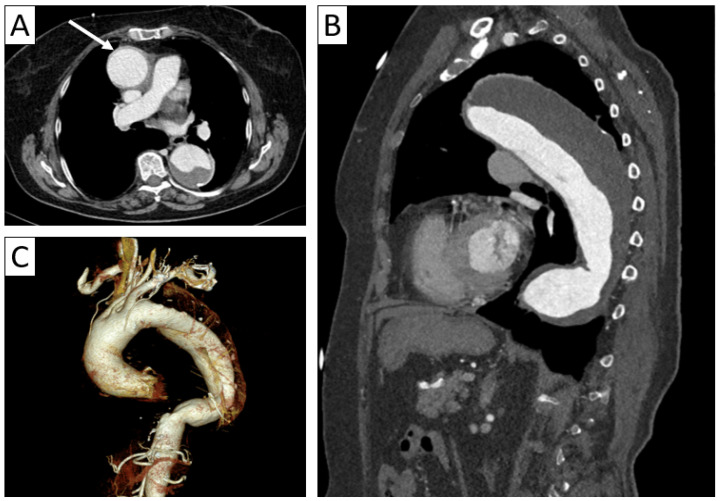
An 80-year-old female patient with giant cell arteritis and rheumatoid arthritis. Axial thoracic CTA (A) with sagittal reformatting (B) and 3D volume rendering (C) demonstrated minimal thickening of the aortic wall (arrow in (A)) and a fusiform aneurysm of the ascending and descending thoracic aorta with parietal thrombosis.
